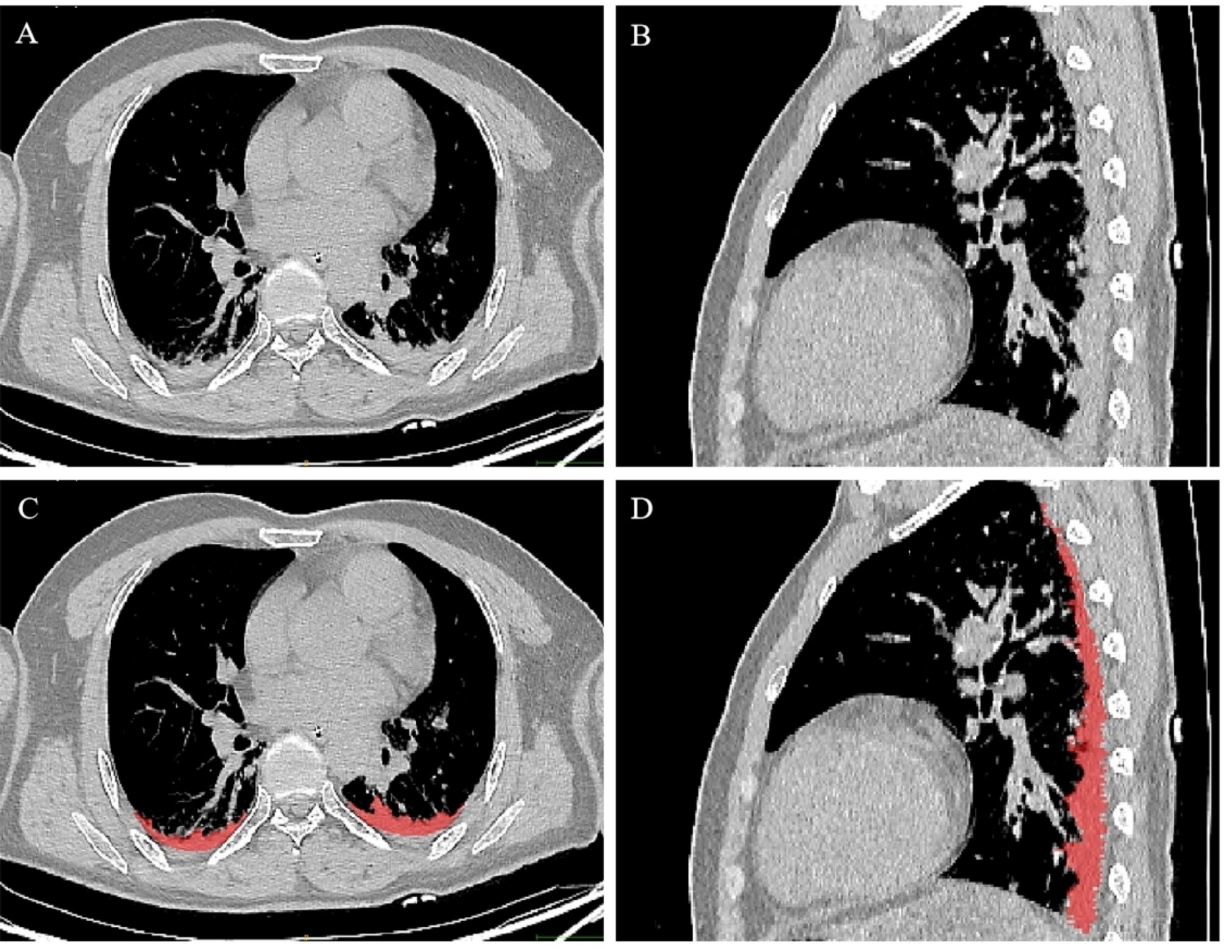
Examples of CT scans. Original chest CT image: (A) transaxial and (B) sagittal scanning. The red part is the atelectasis area marked by ITK-SNAP software: (C) transaxial and (D) sagittal scanning. CT, computed tomography
